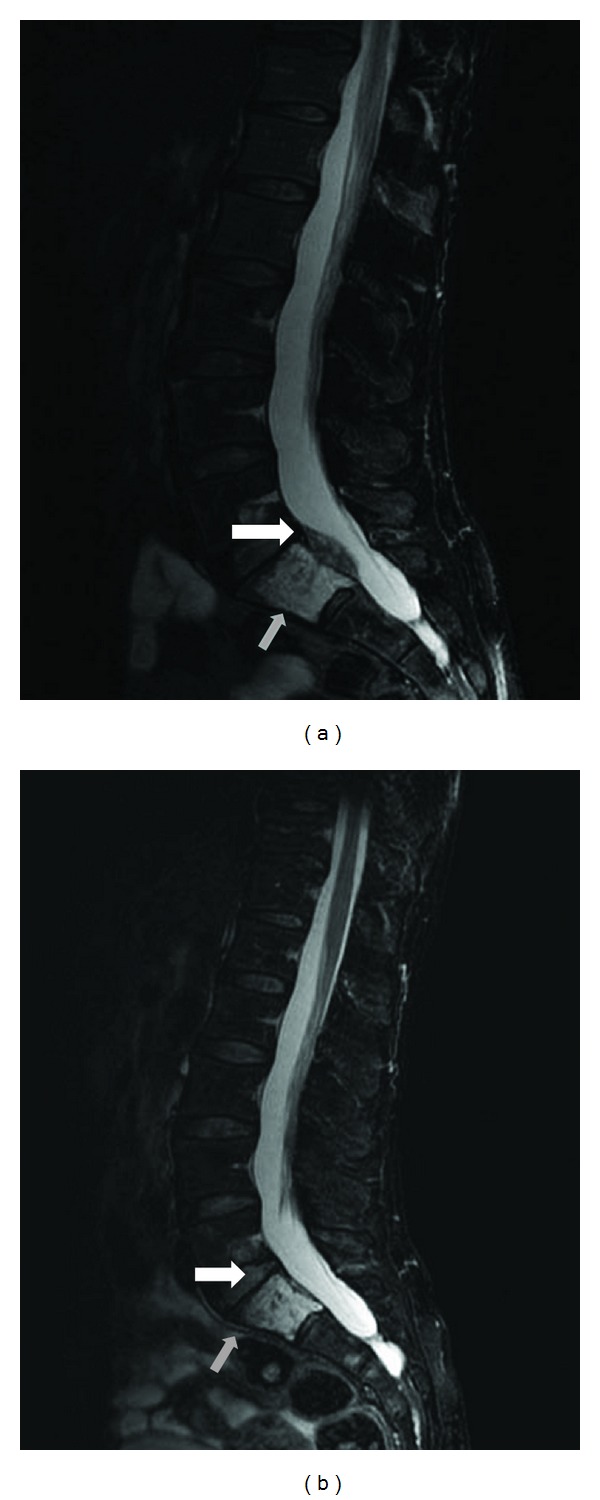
Magnetic resonance imaging (T2 sagittal image) performed at the patient's initial clinical presentation shows S1 metastasis (gray arrow) and cord thecal sac compression (white arrow) (a). Magnetic resonance imaging (T2 sagittal image) performed 3 years after the patient's second RAI treatment shows complete resolution of the tumor (b).
